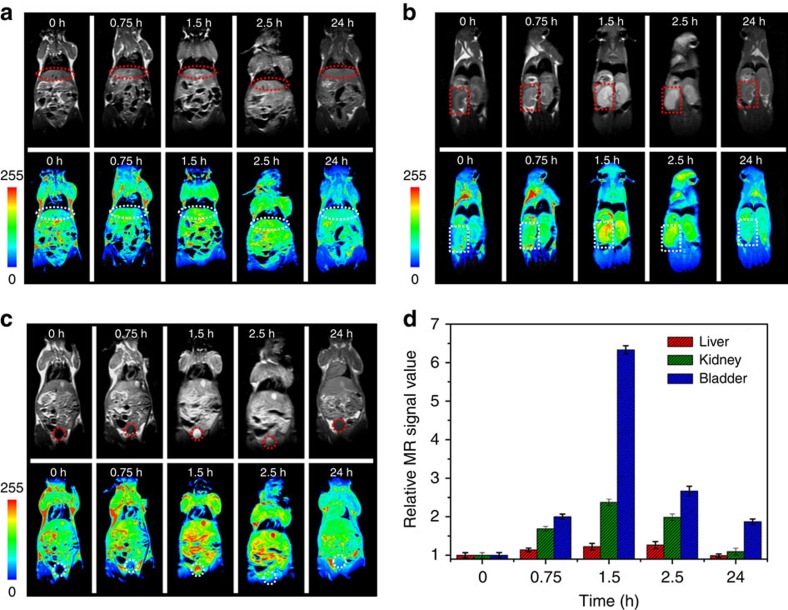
In vivo* biodistribution and clearance of Fe-CPNDs. *In vivo* magnetic resonance images of the (a) liver (ellipses), (b) kidney (rectangles) and (c) bladder (circles) after the intravenous injection of Fe-CPNDs at different time intervals (0 h indicates pre-injection.). (d) Corresponding data analysis of the magnetic resonance measurements. Error bars indicate the s.d. (*n*=5).
